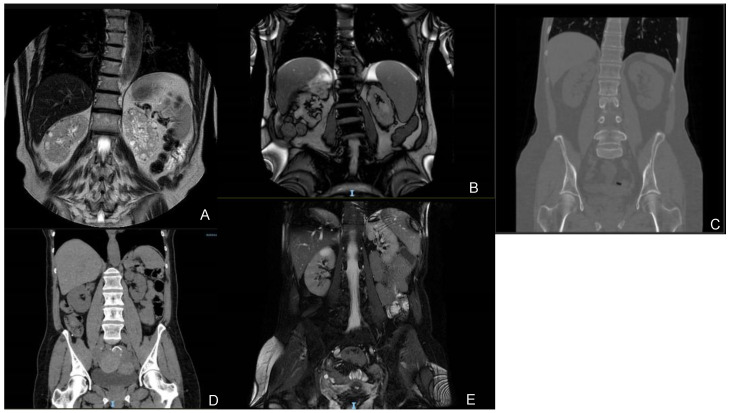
Imaging scans (A–E). (A) Patient 1: MRI (05/2023); (B) Patient 2: MRI (12/2022); (C) Patient 3: CT (03/2023); (D) Patient 4: CT (05/2023); (E) Patient 5: MRI (07/2020).
